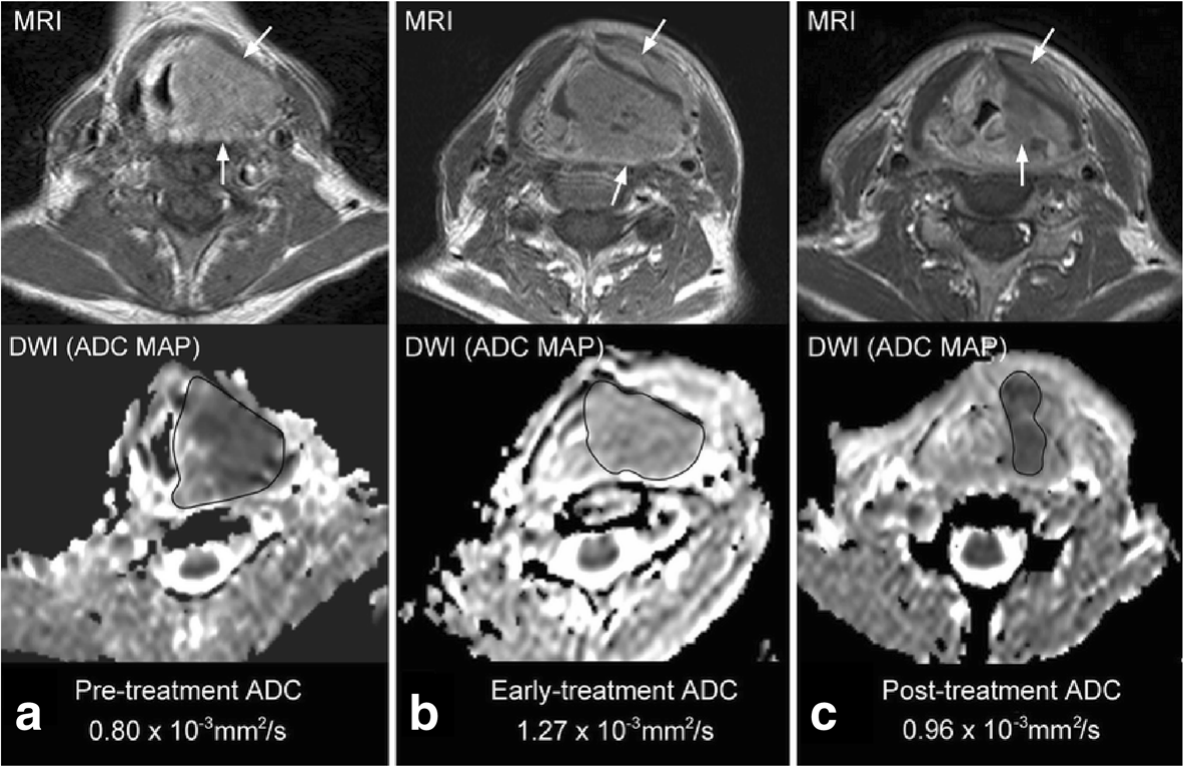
a, b & c. Change in DWI over treatment for prediction of CRT response. ADC maps of a hypopharyngeal SCC (*arrows*) with local failure; a Pre-treatment, b two weeks intra-treatment, c 6 weeks post-treatment. The ADC shows the expected initial early rise in ADC but there is a later fall in ADC (believed to be due to repopulation of tumour cells). {Image reproduced from: King AD, Mo FK, Yu KH, et al. (2010) Squamous cell carcinoma of the head and neck: diffusion-weighted MR imaging for prediction and monitoring of treatment response. Eur Radiol 20:2213-20.}
